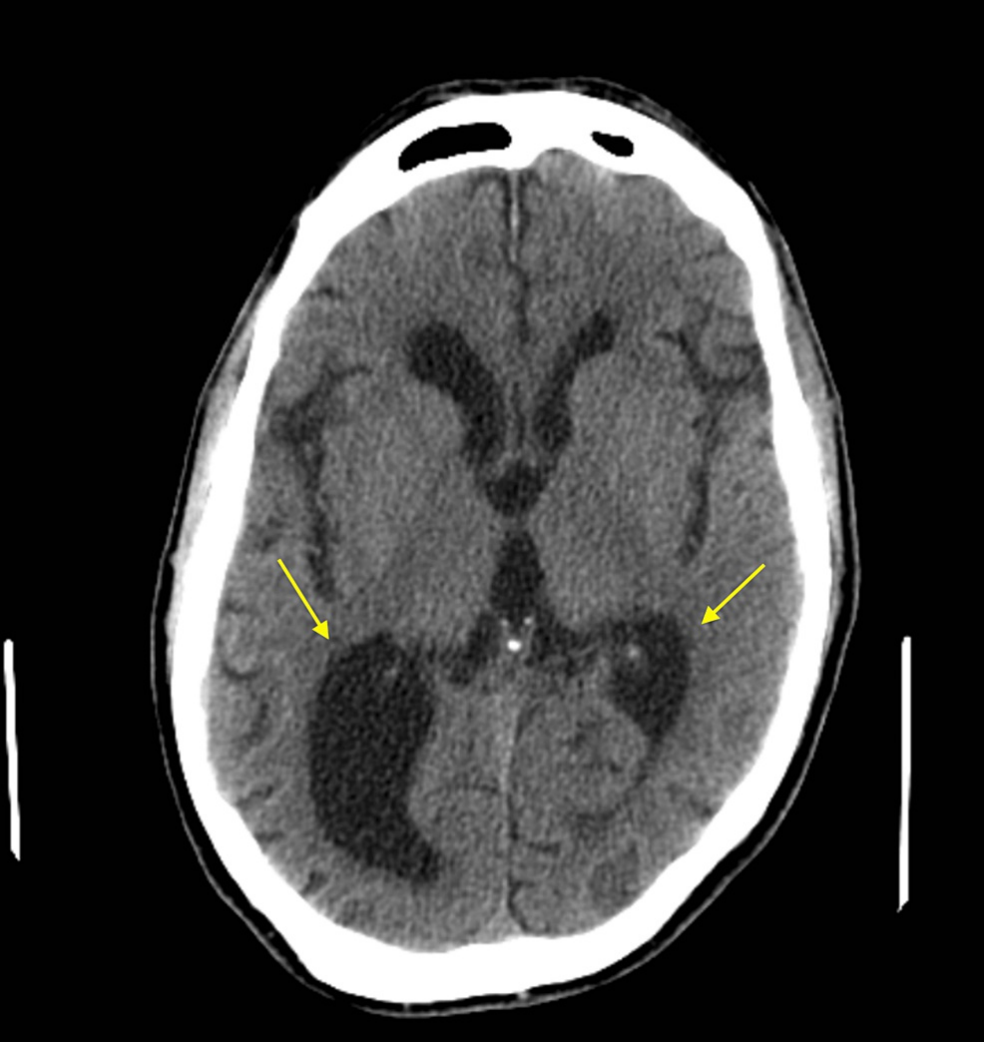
CT scan of the head: axial view. Asymmetric dilatation of the right occipital horn due to agenesis of corpus callosum resulting in colpocephaly.
